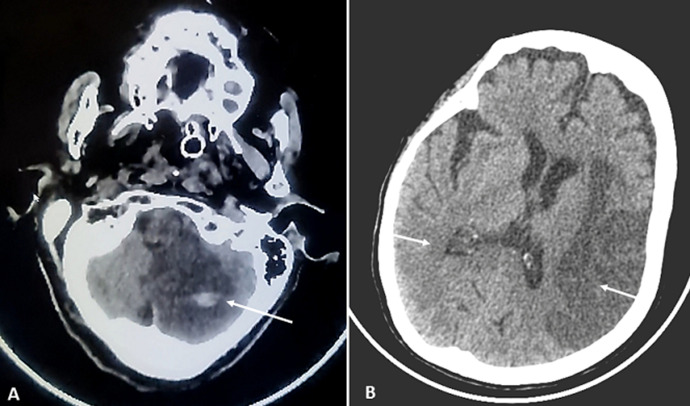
axial head computed tomography shows: A) infarction of the left cerebellar hemisphere with hemorrhagic transformation; B) acute ischemic infarction in the distribution of the right middle cerebral artery and sub-acute left middle cerebral artery territory infarction
